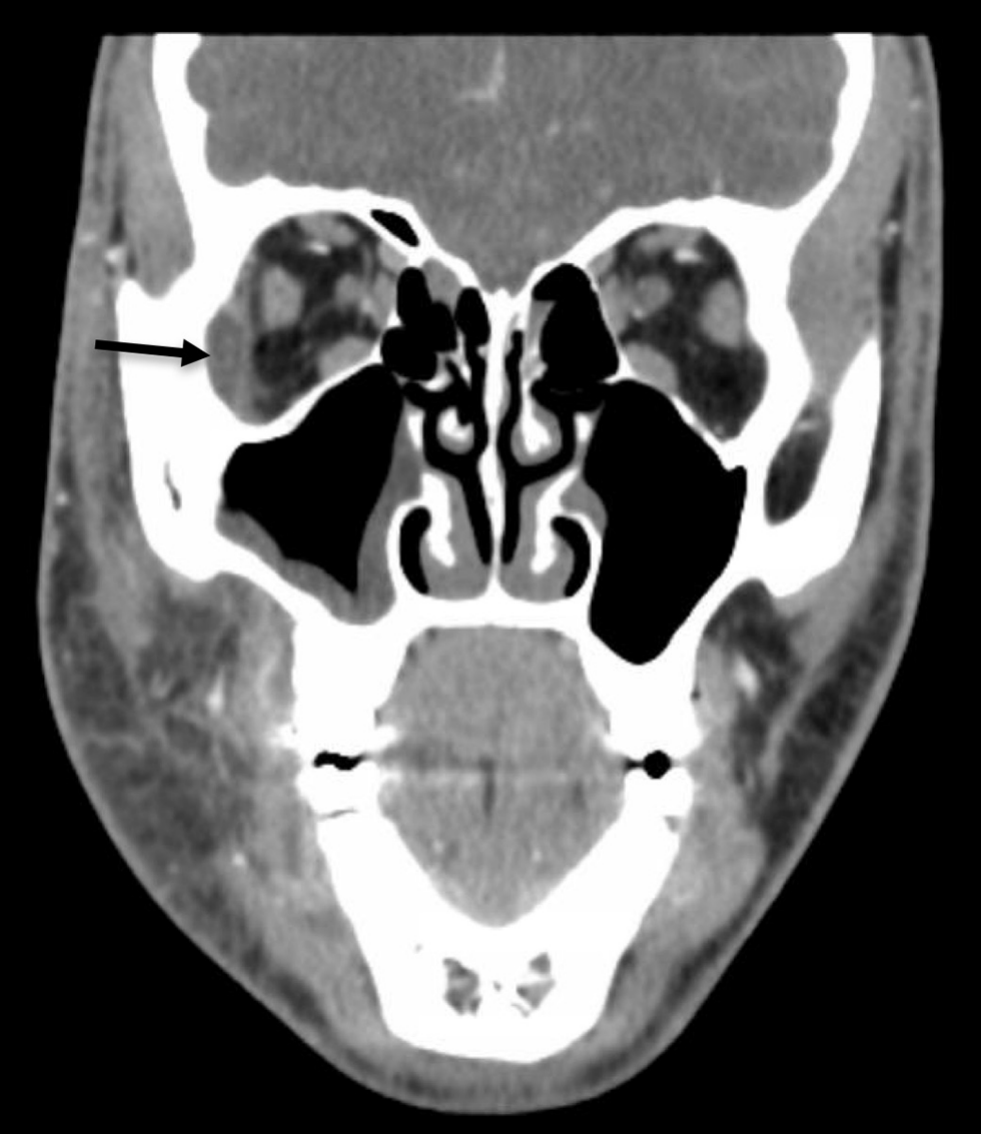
Computed tomography scan coronal section displayed the right lateral orbit subperiosteal abscess (black arrow) and right maxillary sinusitis.
